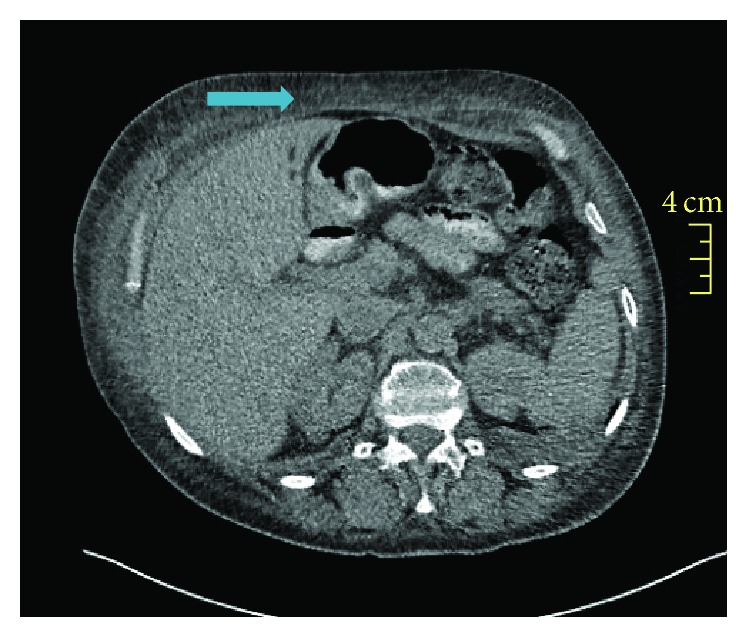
77-year-old female patient with a tongue lesion and heart failure. Computed tomography of the abdomen reveals fat opacification (bold arrow).
